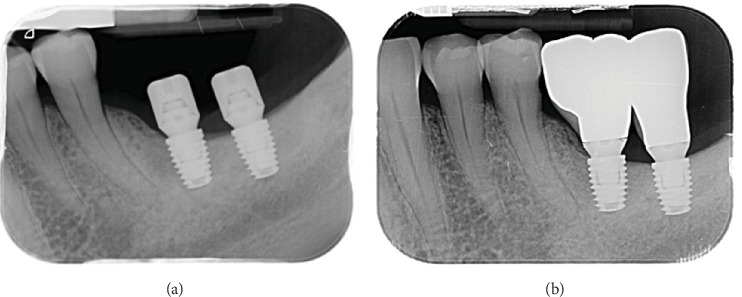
Periapical radiographs performed with paralleling technique and a customized bite jig taken at (a) 3 and (b) 6 months follow-up.
